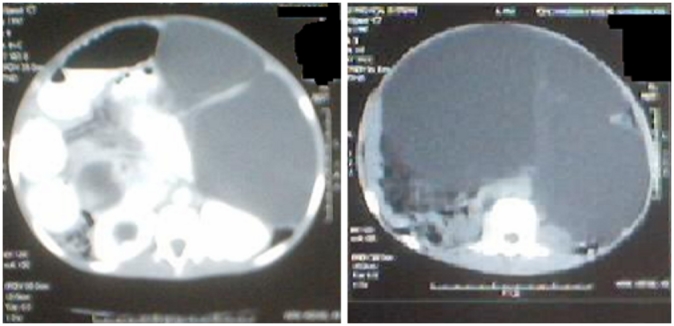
The CT- scan demonstrating a cystic mass
